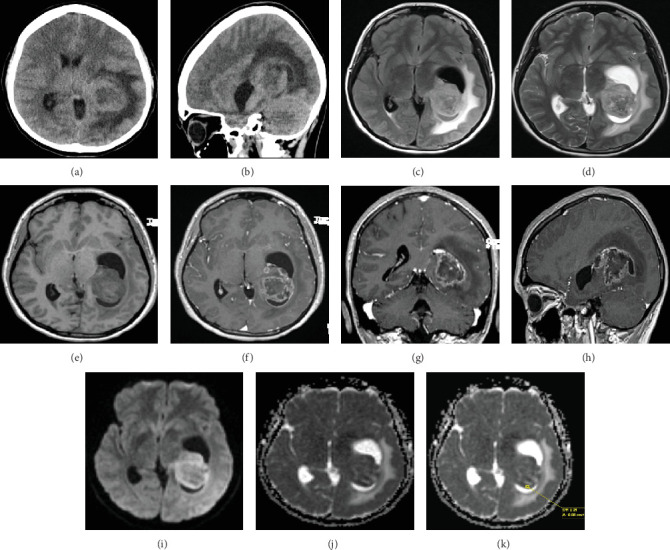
Left intraventricular heterogeneous lesion with temporal horn entrapment. (a, b) A 5-cm heterogeneous intra-axial mass centered in the region of the trigone of the left lateral ventricle. Moderate extent of vasogenic edema. Entrapment of the temporal horn of the left lateral ventricle. There is significant mass effect causing a midline shift of 9 mm and diffuse sulcal effacement. (c, d) FLAIR and T2 MRI images confirm the presence of intra-axial mass exhibiting heterogeneous signal on T2-weighted images causing entrapment of the temporal and occipital horns of the left lateral ventricle. There is a moderate extent of vasogenic edema within the surrounding left temporoparietal region. (e, f) 3D T1-weighted images pre- and postgadolinium. Pre-gad, the tumor exhibits hypointense to isointense signal. Post-gad, the tumor exhibits heterogeneous enhancement. There is thin ependymal enhancement of the surrounding trigone of the left lateral ventricle. (g, h) In coronal and sagittal postgadolinium images, the tumor appears to be arising from the left temporo-occipital region extending into the trigone of the left lateral ventricle. The left choroid plexus is displaced superiorly. There is nodular extension of nonenhancing tumor anteriorly into the left hippocampus, which exhibits volumetric expansion. (i–k) DWI and ADC MRI sequences reveal a heterogeneous diffusion restriction with ADC values reaching as low as 511, indicative of high grade.
